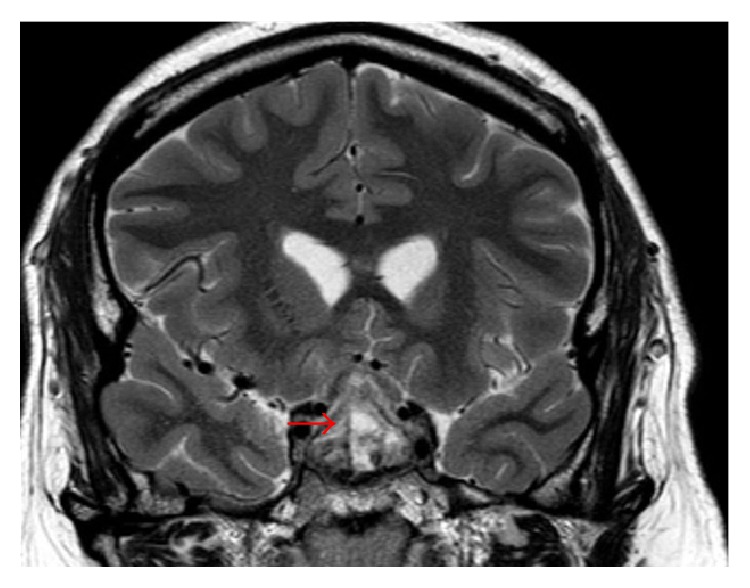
T2 COR SELLA (brain MRI with and without contrast). Red arrow indicating pituitary mass with evidence of internal hemorrhage.
